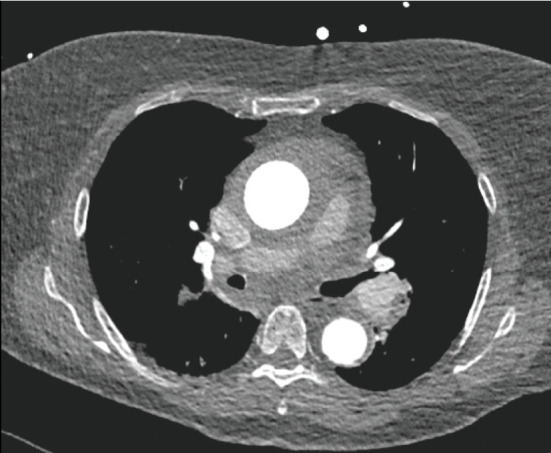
Contrast-enhanced computed tomography of the aorta, performed at our institution, showing a vessel with a normal diameter of 38 mm without visible dissection membrane or aortic wall thickening. The aorta is therefore described as free of pathology.
